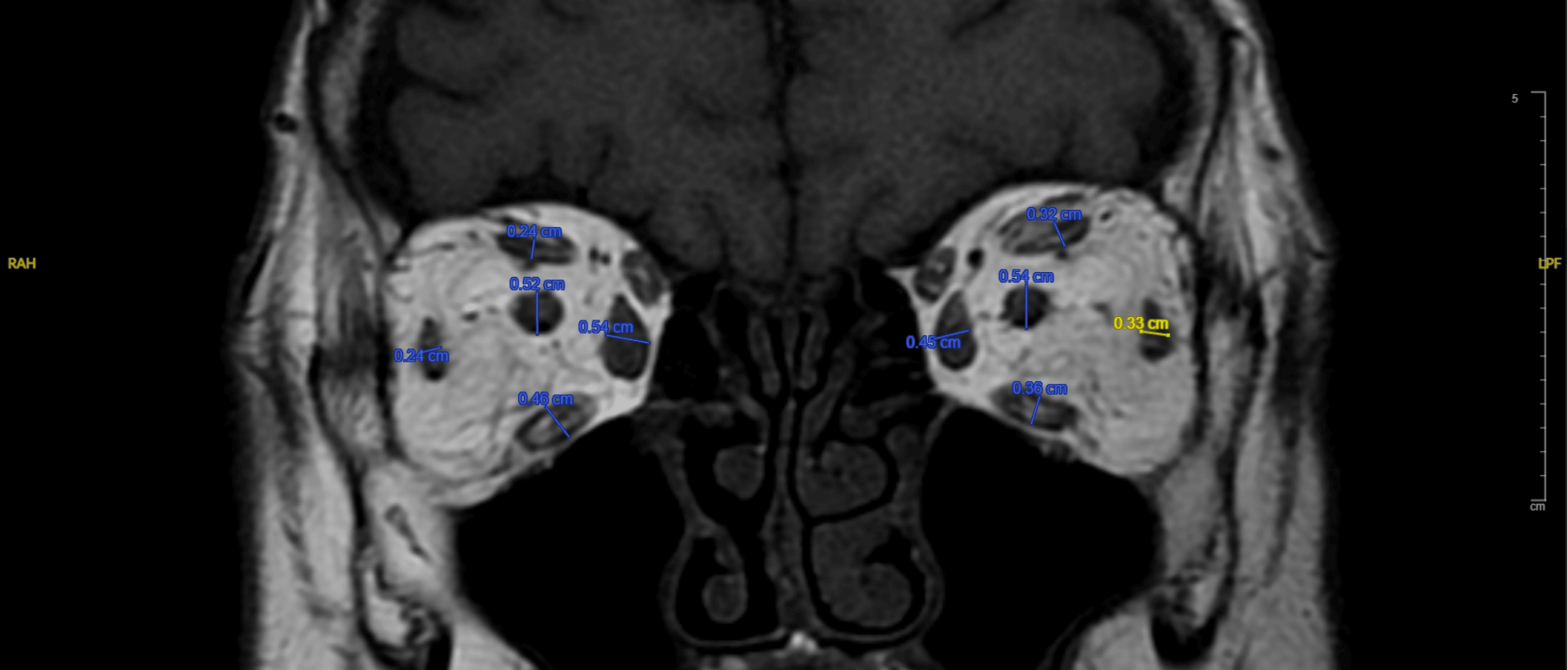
Measurement of extraocular muscles (mm) and optic nerve diameter (mm) on coronal T1WI T1WI: T1-weighted image
